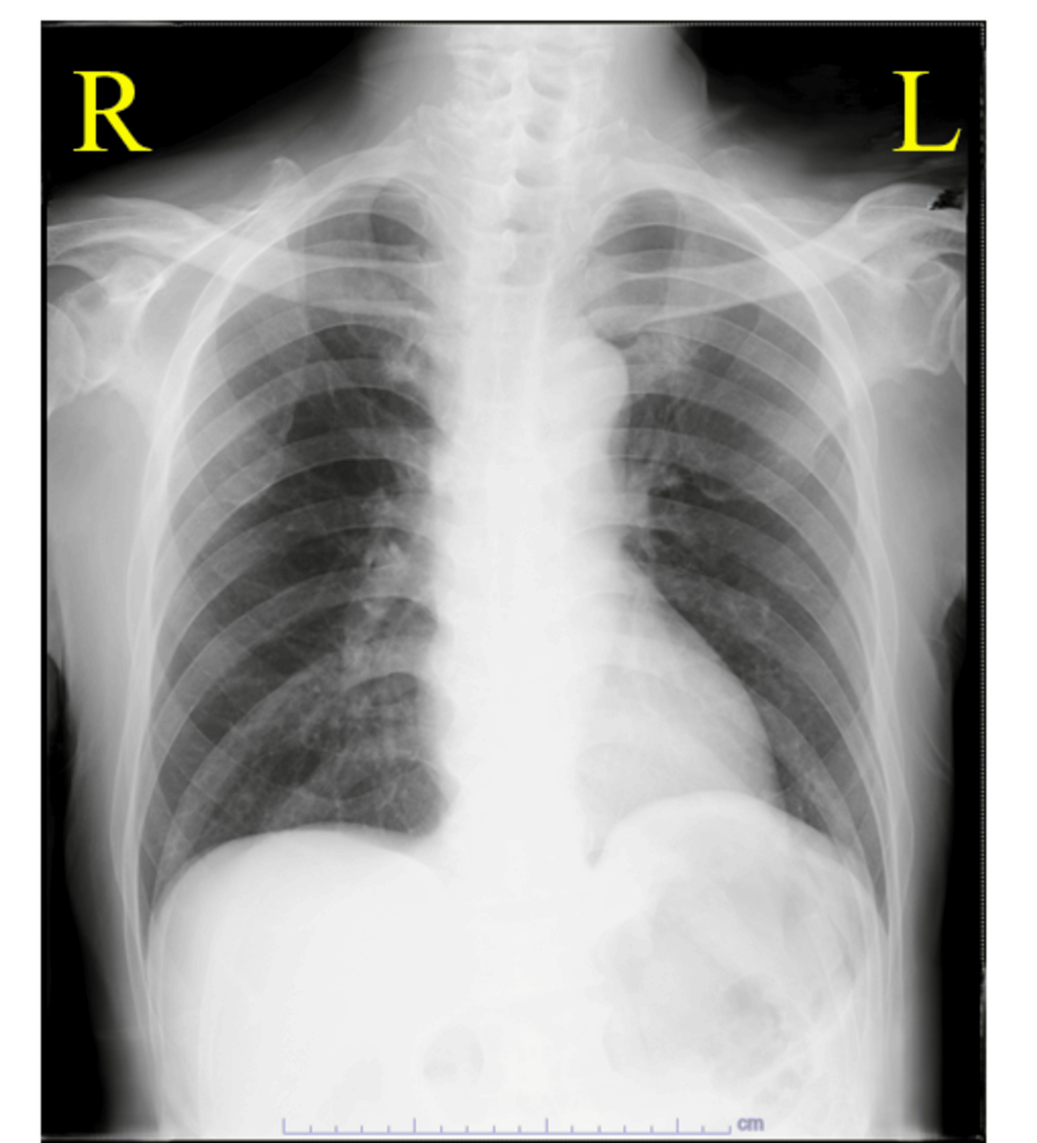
Chest X-ray image at admission
